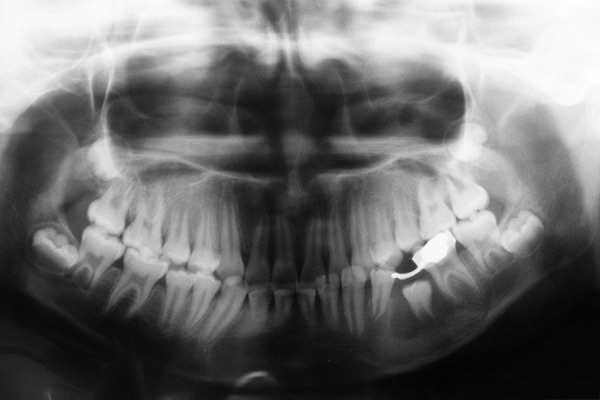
Orthopantomogram after 6 months follow-up showing short band loop space maintainer in relation to 75 and the successive permanent second premolar
